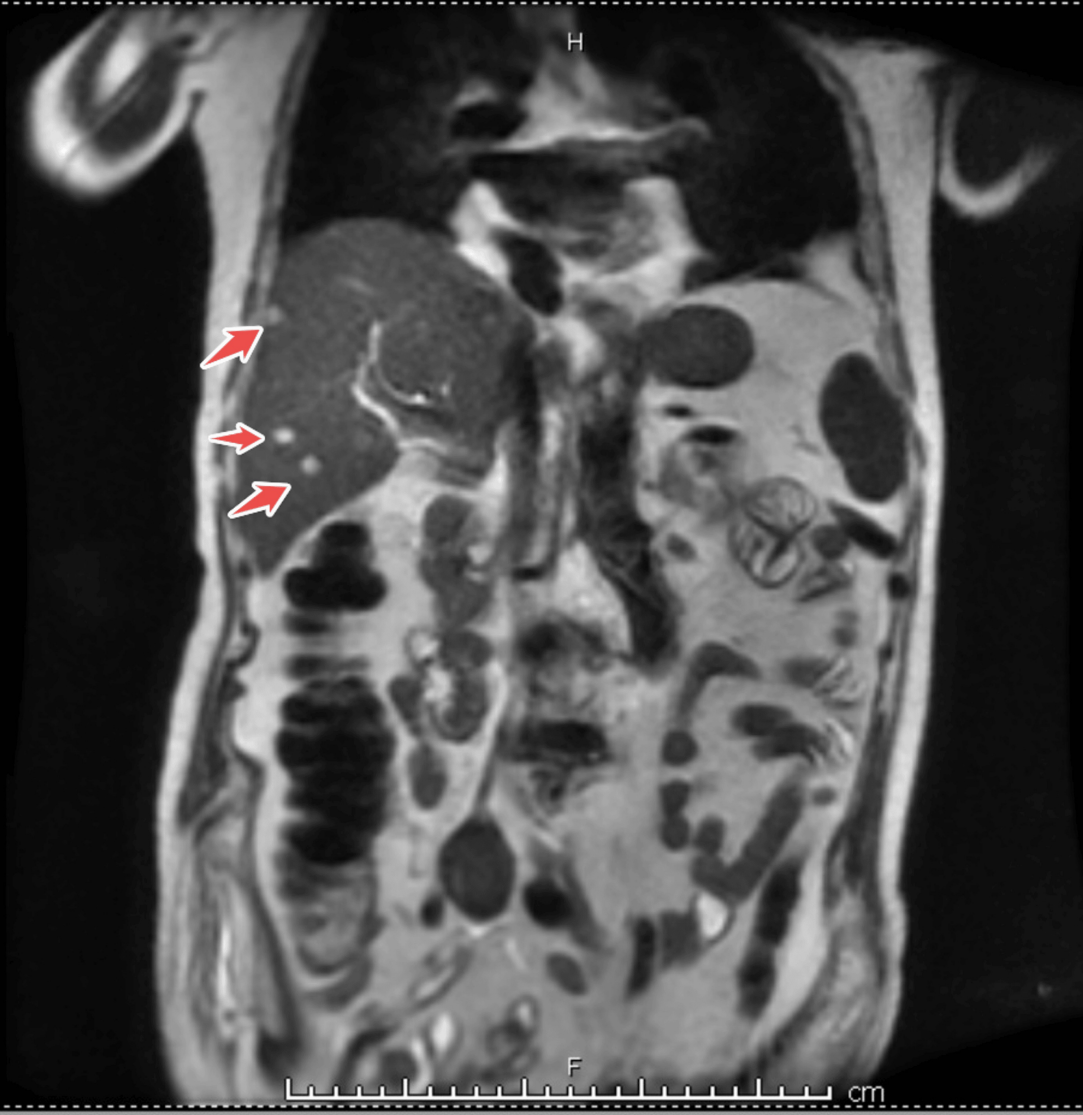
Coronal abdominal MRI demonstrates multiple focal hepatic lesions (highlighted by arrows) MRI, magnetic resonance imaging
